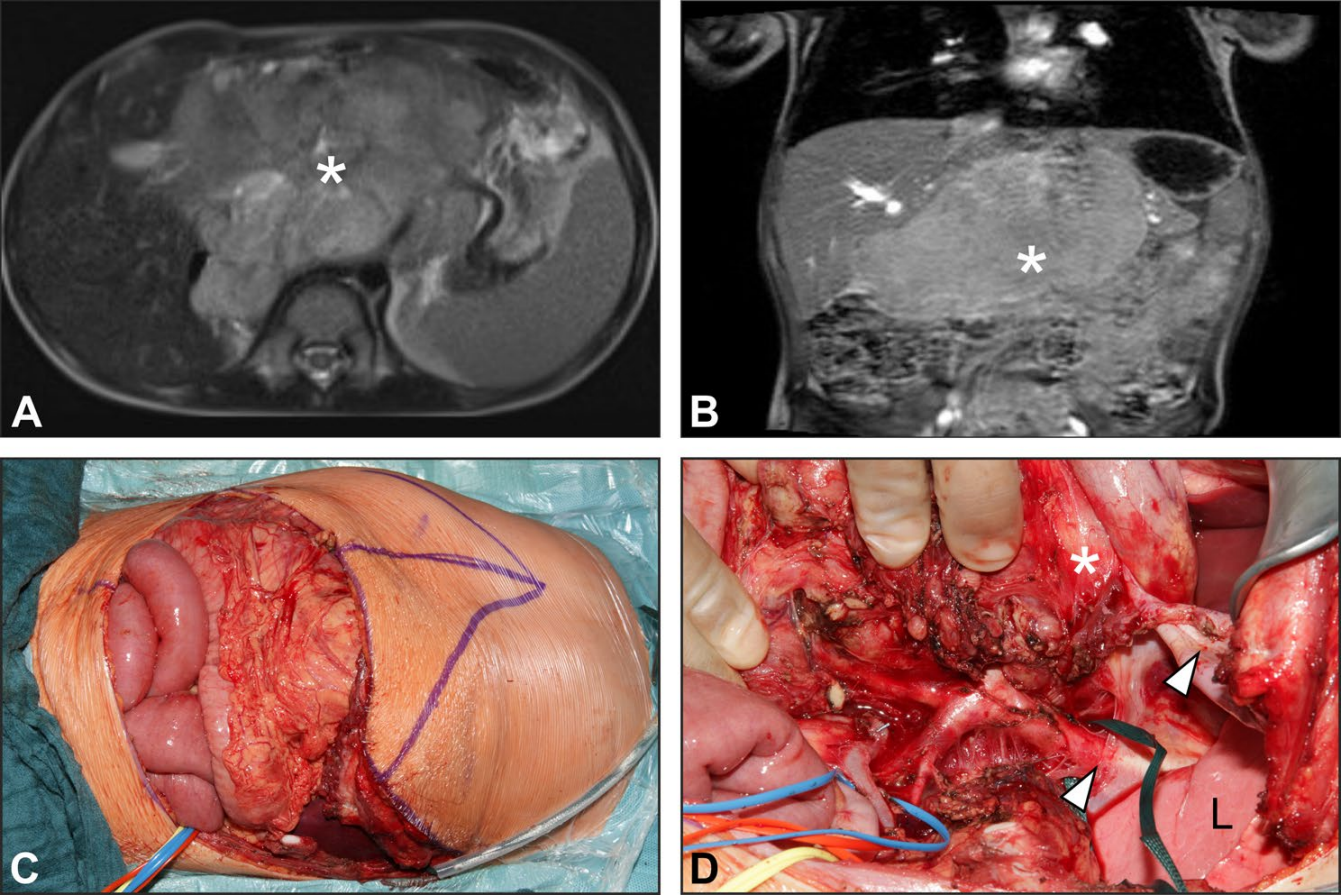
MRI scan of a patient with upper abdominal ganglioneuroma (A, B) suitable for a thoraco-abdominal approach (B), which allowed an adequate exposure of the involved structures (C). Lower arrow: abdominal aorta; upper arrow: left hemidiaphragm; L: lung. *Tumor mass
